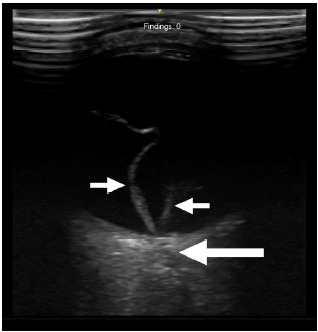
Point-of-care ultrasound demonstrating retinal detachment. The small arrows denote the retina having detached from the posterior globe. The large arrow denotes the optic nerve sheath.
